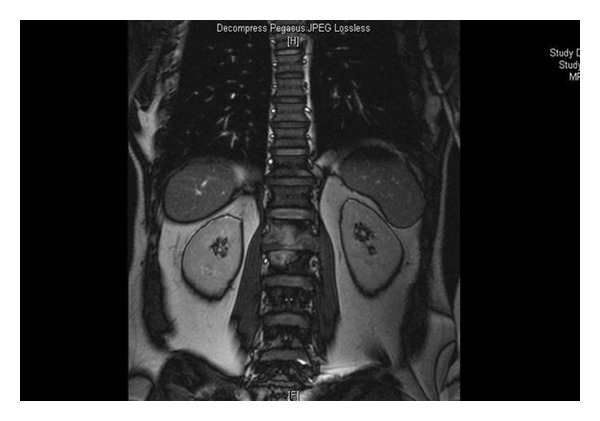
Magnetic resonance imaging of pathologic signalization in lumbar vertebrae.
